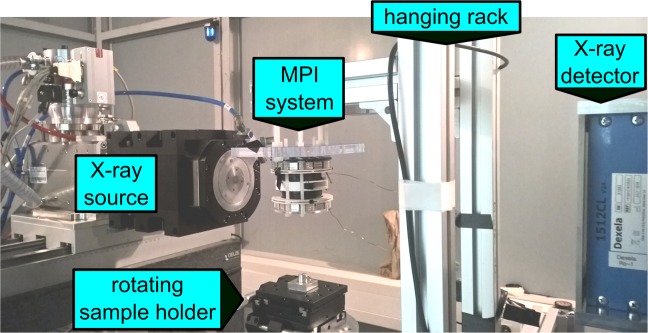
Image of the MPI-CT system: left the X-ray source, in the center the MPI scanner and on the bottom the rotating sample holder.
